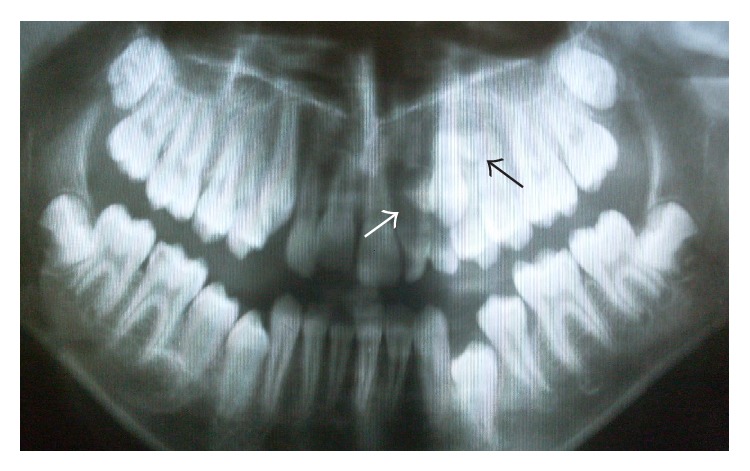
Two-year follow-up radiograph showing 2 additional supernumerary teeth, 1 in the maxillary left premolar region and 1 in the maxillary anterior region.
